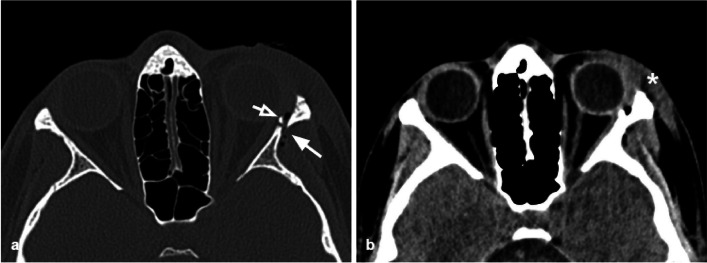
Axial CT acquisition with bone (a) and soft tissues (b) algorithms of a pure fracture of the left lateral orbital wall (white arrow). Slightly displaced fracture of the lateral orbital wall with adjacent intraorbital small air bubbles. A small bony fragment (empty arrow) presents contact with the lateral rectus muscles, that preserves regular morphology and thickness. Hematoma of the subcutaneous soft tissue (asterisk)
